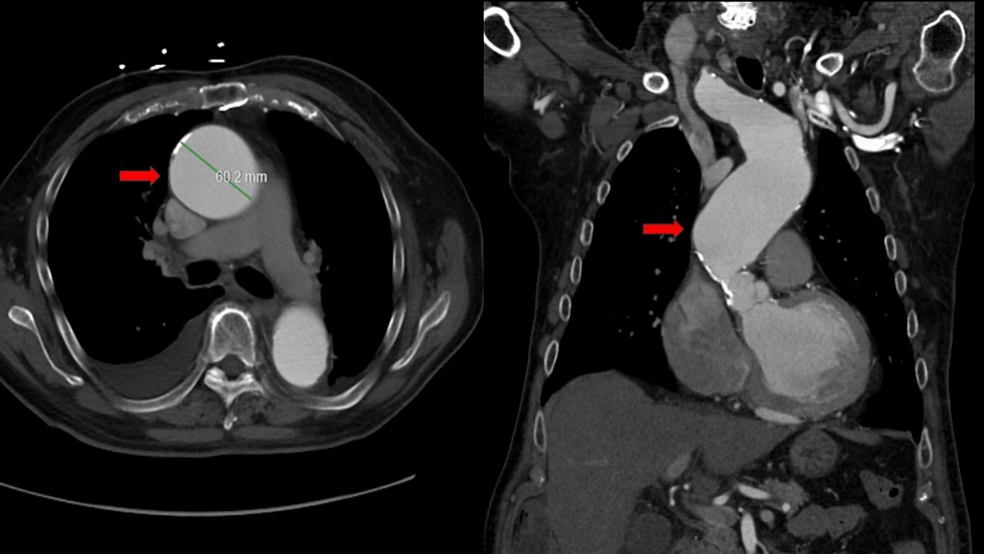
CTA chest showing thoracic aortic aneurysm measuring 6 cm and not involving aortic arch or arch vessels (red arrow pointing toward aneurysm) CTA, computed tomography angiography
